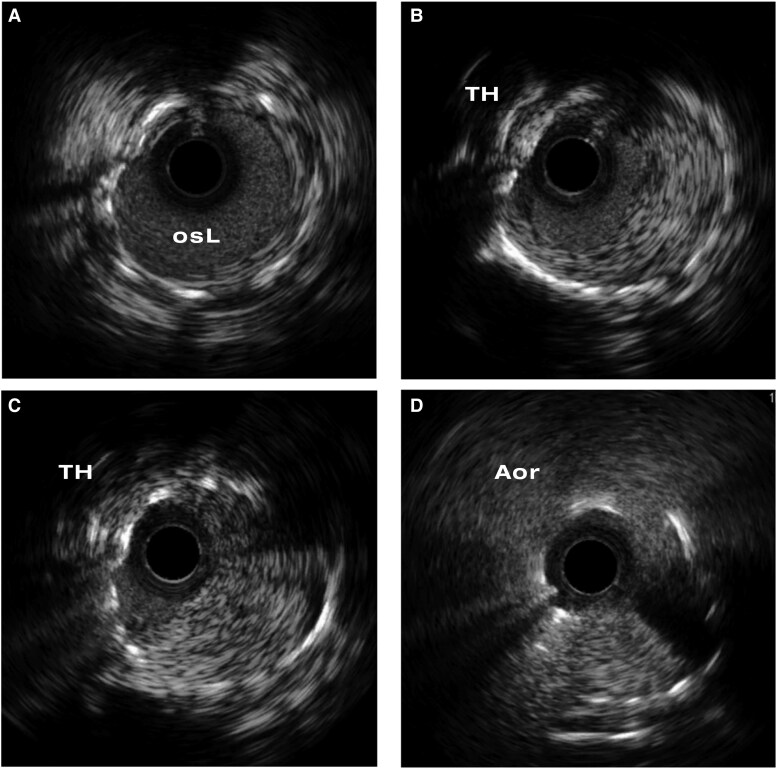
Intravascular ultrasound of chimney stent in-stent restenosis. Patent stent inside of left main and ostium (*A*). Neointima formation at chimney stent out of left main with contralateral compressive deformity by transcatheter heart valve stent (*B* and *C*). No stenosis at the ostium of chimney stent (*D*).
